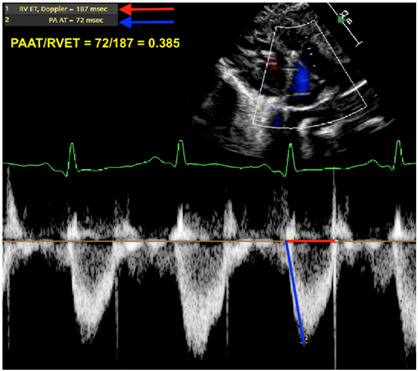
Legend: The pulmonary artery acceleration time to the right ventricular ejection time is a ratio providing some insight on the RV afterload. In situations where the RV afterload is increased (either due to high pulmonary vascular resistance or other contributors—flow/pressure transmission), this ratio decreases. The pulsewave Doppler envelope of the RV outflow tract shifts from a parabolic shape to a more triangular shape with a steeper diastolic upstroke. Here, the ratio is 0.385 (abnormal < 0.31; some use a cutoff of <0.25).
